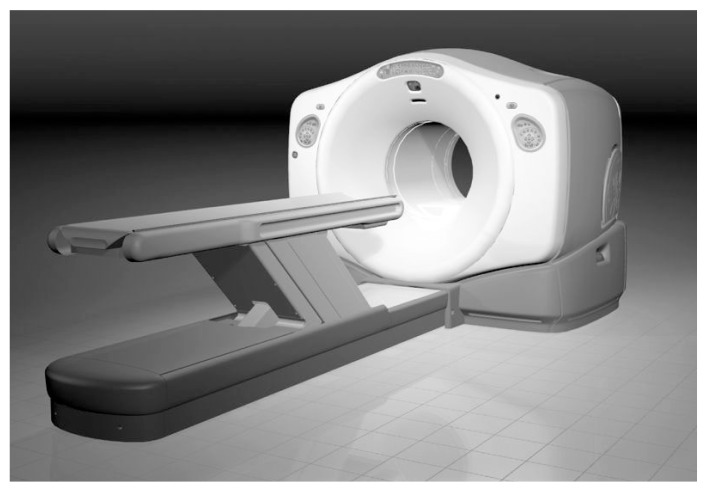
This state-of-the-art scanner is used to obtain both PET and CT images. SOURCE: Photograph provided courtesy of Dr. Alexander Y. Tokman, General Electric (GE) Medical Systems, Milwaukee, Wisconsin.
